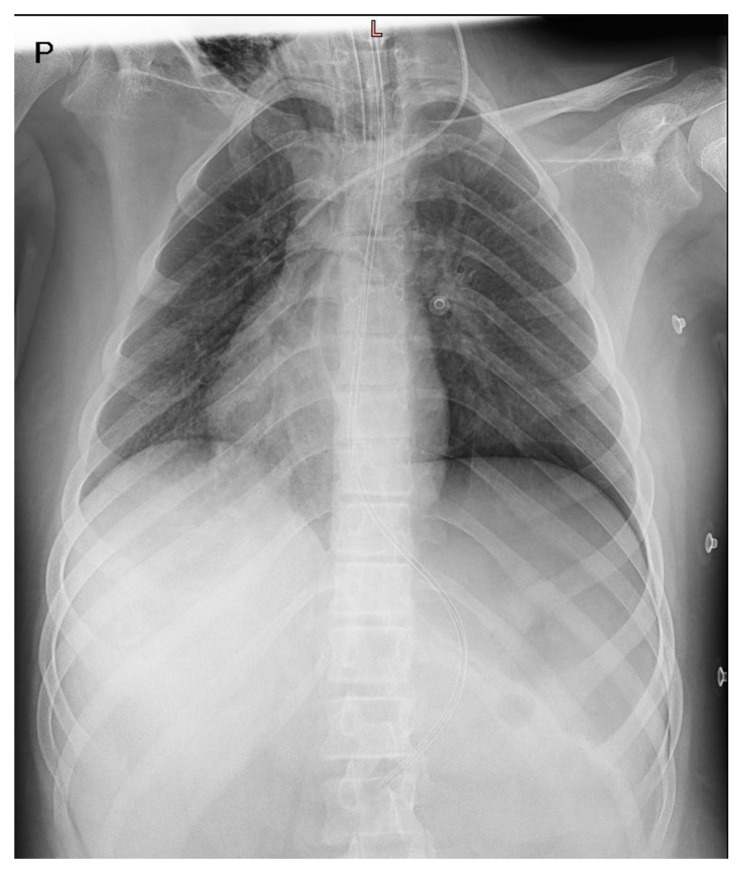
Massive atelectatic and interstitial edematous changes, along with a left pneumothorax and features of mediastinal emphysema in chest X-ray.
